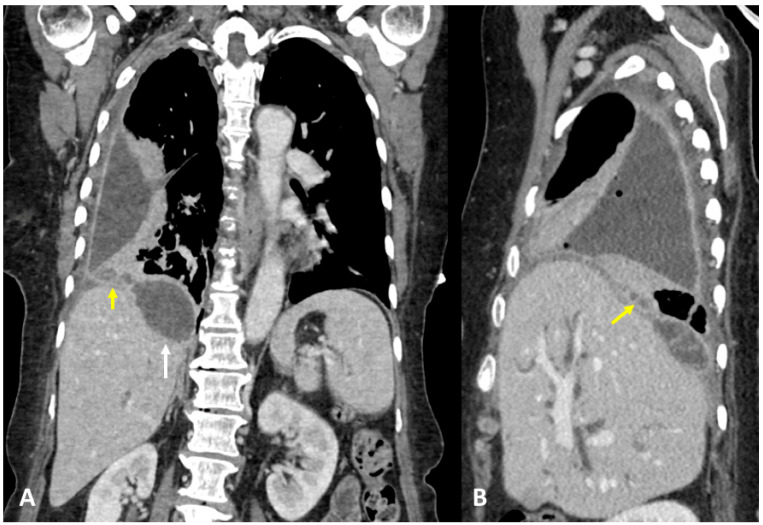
(A,B) Contrast-enhanced CT and coronal (A) and sagittal planes (B) demonstrate a right pleural empyema with gas bubbles and a collection (white arrow) located between the diaphragm and the liver. (A) Definite fistulous tract is noted (yellow arrows).
